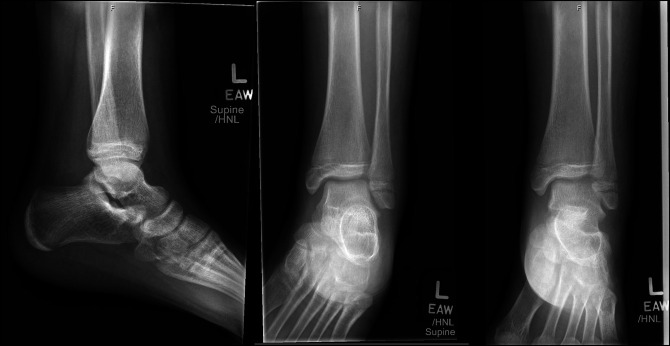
AP, mortise, and lateral view ankle radiographs 2 weeks after the injury demonstrating maintained alignment with no fracture displacement.
